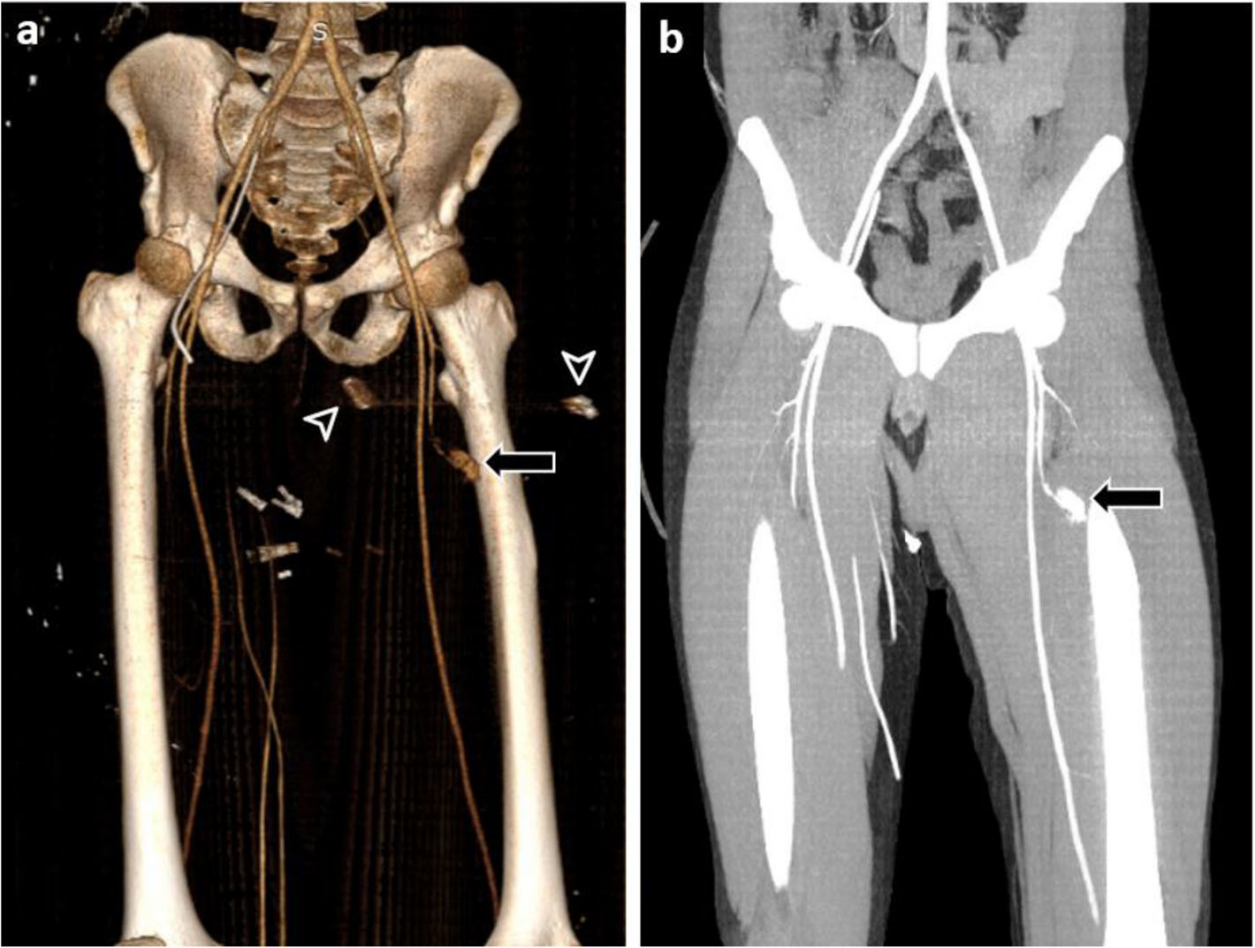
Computed tomography angiography images of a patient with arterial injuries due to bomb fragment. a-b Volume-rendered three-dimensional reconstruction and coronal MIP images showing pseudoaneurysm (arrows) and bomb fragment (arrowheads) in the profunda femoris artery. There is no bone fracture in this patient
